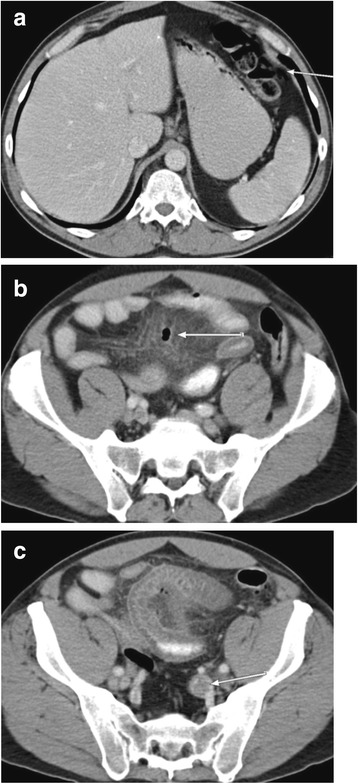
54-year-old man with CLL on maintenance with Rituximab with new onset pain abdomen. a Axial CT images of the abdomen obtained during portal venous phase show small focus of air under the left hemidiaphragm (white arrow). b Axial CT images of the pelvis obtained during portal venous phase show a focus of air in the mesentery (white arrow) (c). Axial CT images of the pelvis obtained during portal venous phase demonstrate thickening of the small bowel loop with a filling defect in the left internal iliac artery (white arrow). Findings were compatible with small bowel perforation
